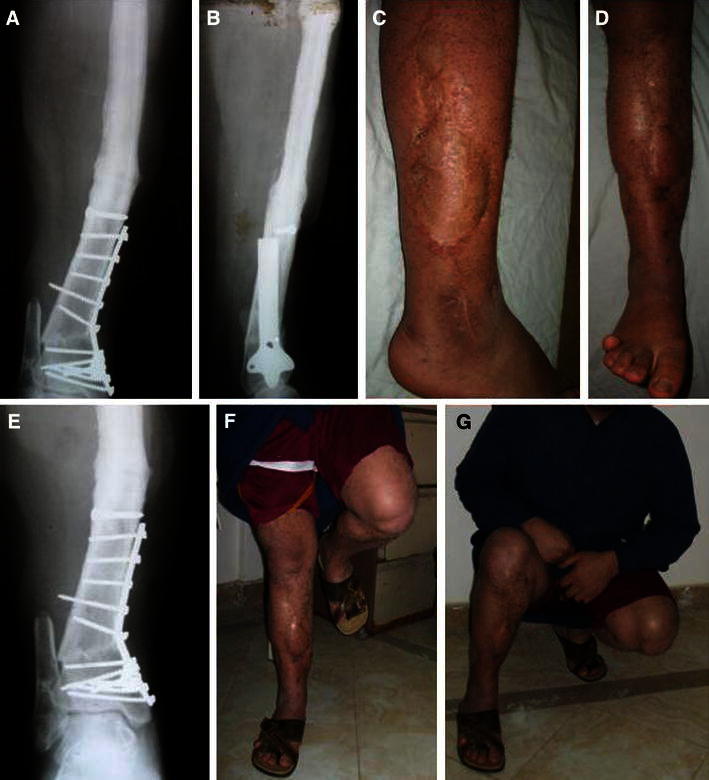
Clinical and radiological outcome of 25-year-old male patient three years after the operation. a, b Plain X-ray taken six months after supramalleolar osteotomy for valgus angulation (note also the hypertrophy of the fibula); c, d photo of the leg after skin healing of incisions and flaps; e X-ray taken one year after the osteotomy, showing good union; f, g photos showing weight bearing and movement of the patient at the end of the follow-up period
